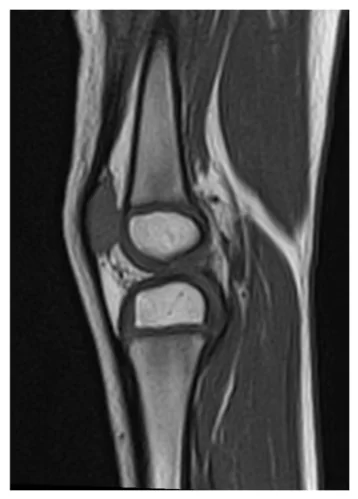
MRI of the left knee demonstrating ACL and PCL deficiency.
